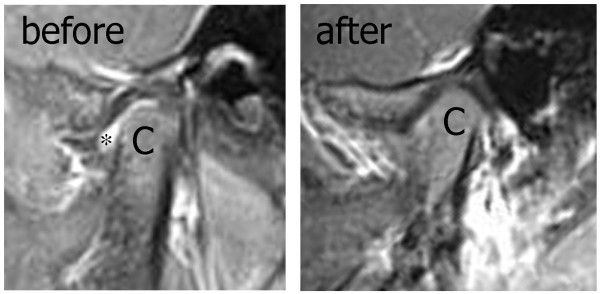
Post-contrast, fat-saturated MRI images of an arthritic TMJ in a child with JIA before (left) and after (right) intra-articular corticosteroid injection. The head of the condyle (C) and intra-articular fluid (*) prior to the injection are noted.
